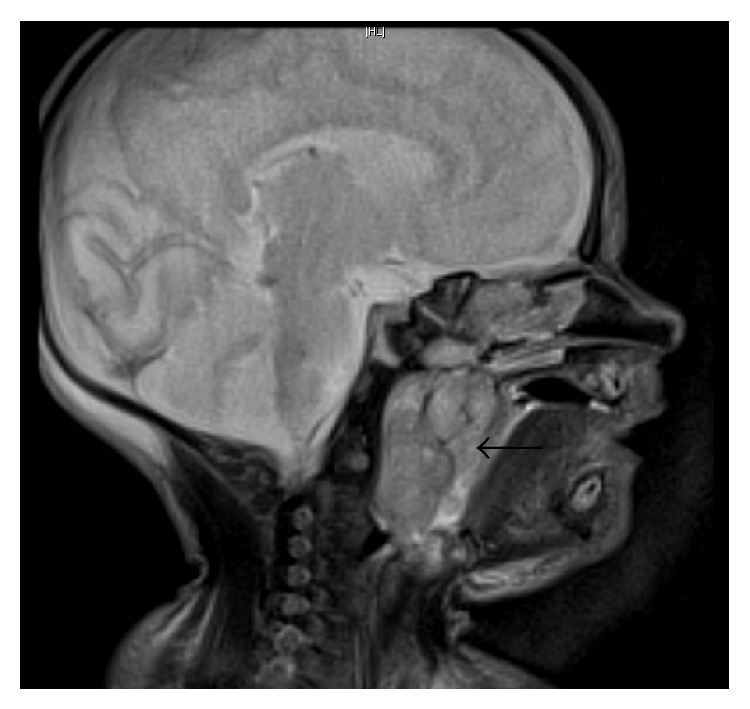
T2 weighted MRI of NPT (sagittal view).
